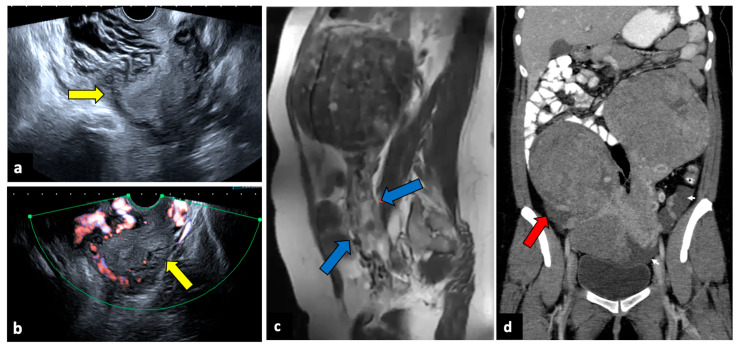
Torsion of a leiomyoma in a 36-year-old woman with acute abdominal and pelvic pain. US (a,b) shows a normal uterus (yellow arrows) surrounded by multiple dilated vessels. In a previous MR (c), a large pedunculated leiomyoma was observed (blue arrows). In a CT scan (d), two pedunculated fibroids are seen; the right one is relatively hypodense, with free fluid in the paracolic gutter (red arrow). Surgery findings confirmed the diagnosis of leiomyoma torsion.
